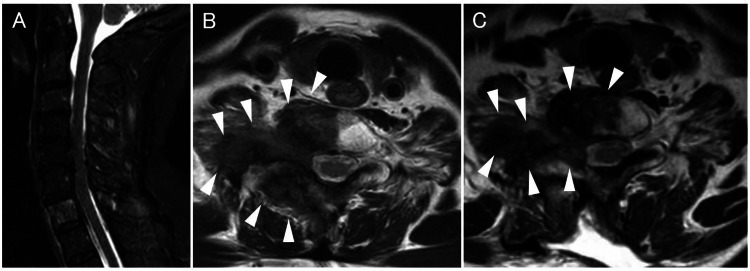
Cervical MRI with a STIR sequence and T2-weighted imaging before and after surgery (A) Cervical MRI with a STIR sequence showing a hyperintense lesion involving the C7 vertebral body and spinous process. (B) T2-weighted axial imaging demonstrating a hypointense space-occupying lesion (arrowhead) involving the right lateral mass of C7 and C7-T1 foraminal space. (C) Postoperative T2-weighted axial imaging showing partial resection of the tumor and residual tumor in right C7-T1 foraminal space (arrowhead). Though postoperative T2-weighted axial imaging is not very different from preoperative T2-weighted axial imaging, her right upper limb pain disappeared and her finger extension muscle strength improved one week after surgery. MRI, magnetic resonance imaging; STIR, short inversion time inversion recovery.
